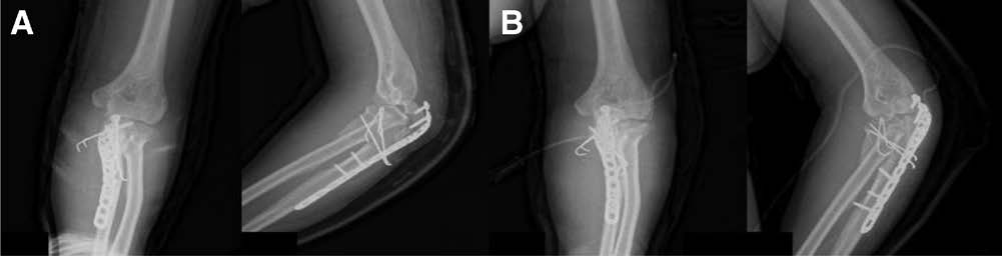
(A) Immediate postoperative X-ray after first operation. (B) Postoperative X-ray after revision.
